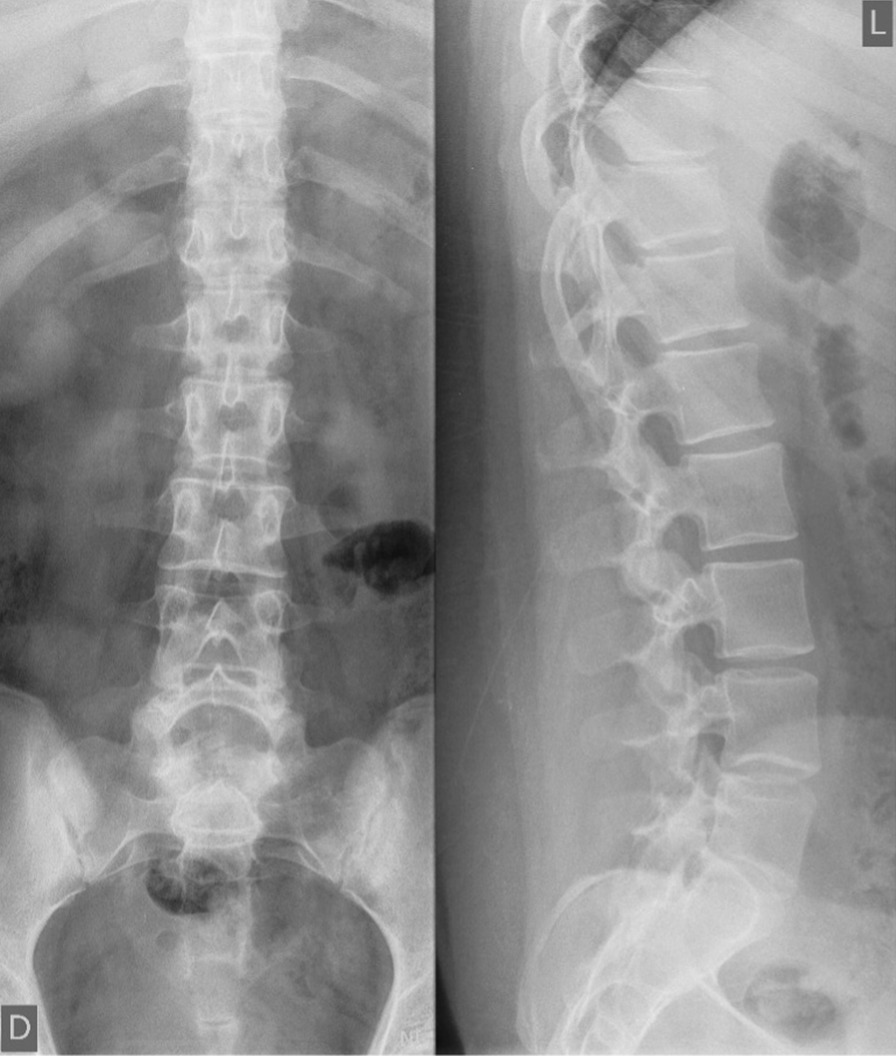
Lumbosacral radiograph—anteroposterior and lateral views. The radiograph of the lumbosacral spine did not show any fractures, which might occur with the patients’ prolonged corticosteroid therapy
